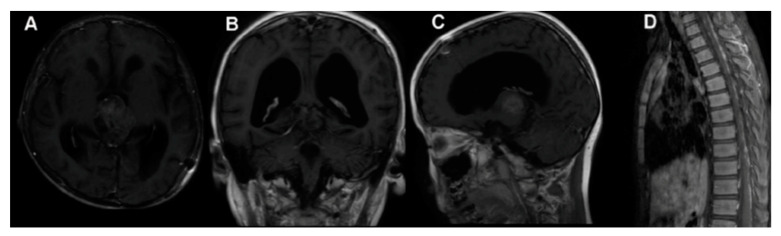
Pineoblastoma MRI. Preoperative MRI (A–C), MRI 1.5 months after surgery showing spinal metastasis (D). Reprinted with permission from Huo et al. (2020) [79]. Dove Medical Press—2021.
